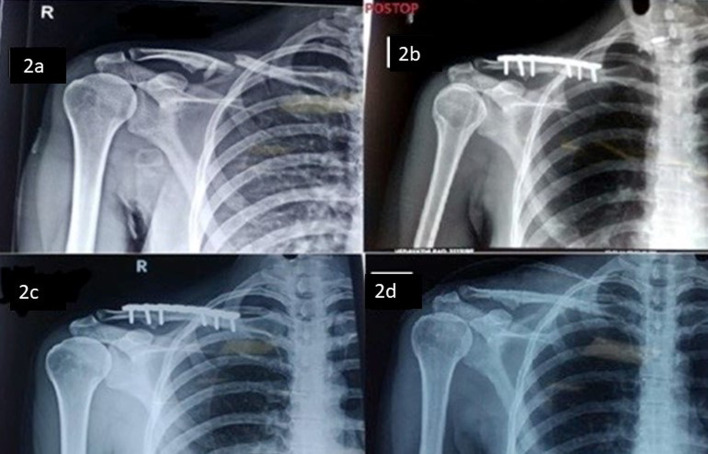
(a) Clavicle fracture, (b) postoperative x-ray after 3 months, (c) fracture union with good results and (d) after implant removal at 1 year.
